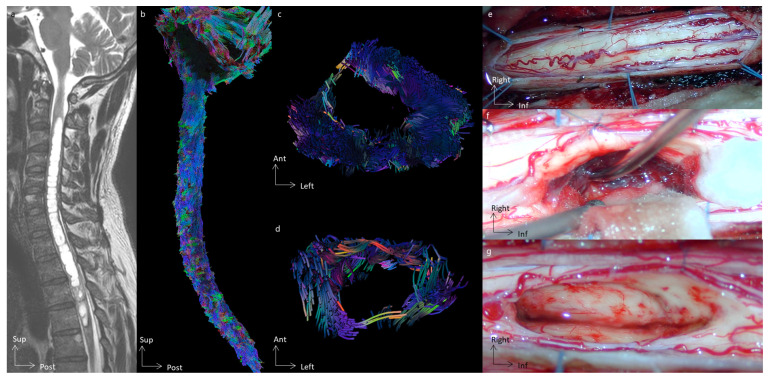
Spinal cord tractography in patient 2, with an ependymoma. (a) Sagittal T2-weighted image showing an intramedullary tumor at the T3 spine level, surrounded by a voluminous syrinx extending up to C3. (b) Sagittal view of the spinal cord tractography with 3D rendering. (c) Axial view of spinal cord tractography at the level of the syrinx (C5 level). (d) Axial view of spinal cord tractography at the tumor level (T3 level). (e) Operative view of the spinal cord before the opening of the median dorsal sulcus. (f) Operative view once the spinal cord is open and dorsal tracts gently retracted, allowing for a safe removal of the tumor. A cleavage plane was found, and as demonstrated by the 3D tractography rendering, the tumor did not invade the spinal cord. (g) Operative view demonstrating a gross total resection of the tumor.
